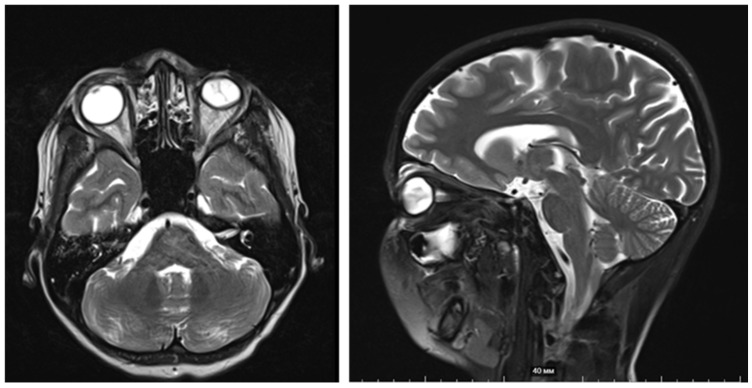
MRI of the orbits and brain (March 2025, 16 y.o.) after radiotherapy and 3 months of immunotherapy (5 infusions): decreased soft-tissue tumor mass.
